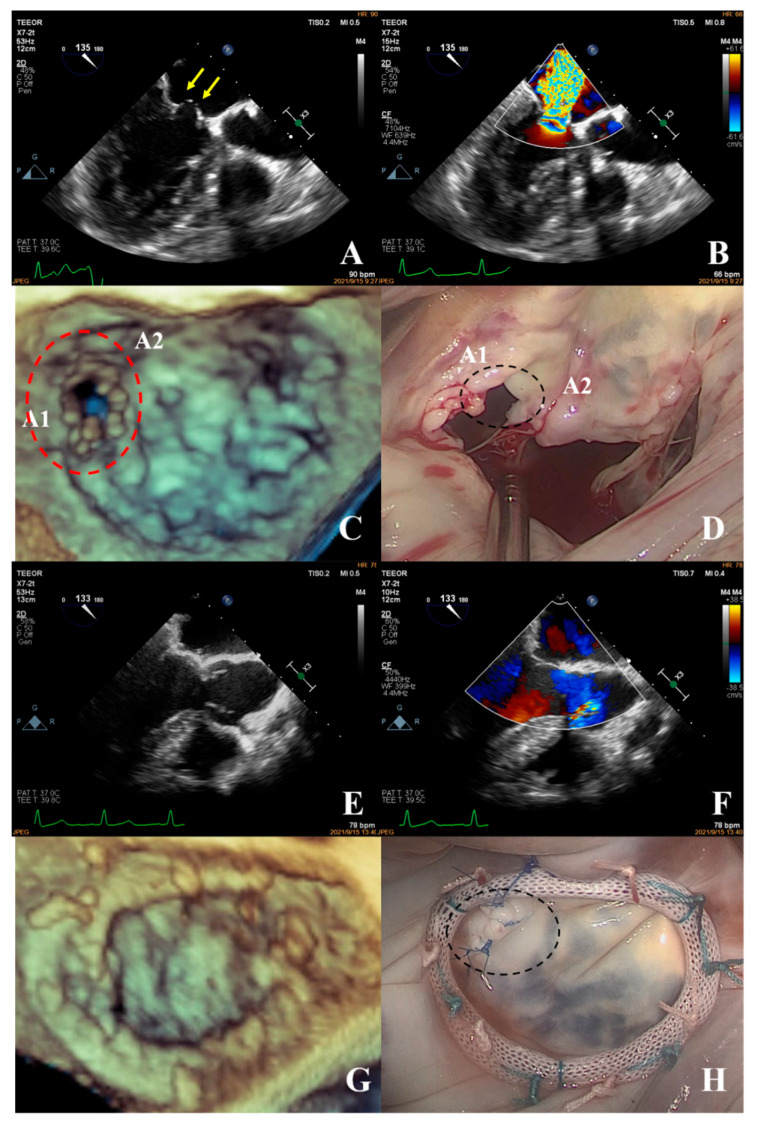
Prolapse of the anterior mitral valve with apparent discontinuity (A) (yellow arrow shows the cleft of the leaflet), massive regurgitation during systole, VC: 8.0 mm (B); the three-dimensional image of the mitral valve shows a cleft (C) between the A1 and A2 segments (dotted red line). During surgery, a cleft (D) was observed between the A1 and A2 segments of the anterior mitral leaflet, consistent with the preoperative diagnosis. The cleft was sutured and the mitral valvular ring (H) was placed. The surgical technique score was 1 + 1 = 2. After the spontaneously returned heartbeat, the forming effect was good and no obvious reflux signal (E,F) was found. The annuloplasty ring of the mitral valve was visible on 3D ultrasound (G), which was consistent with the intraoperative view (H).
